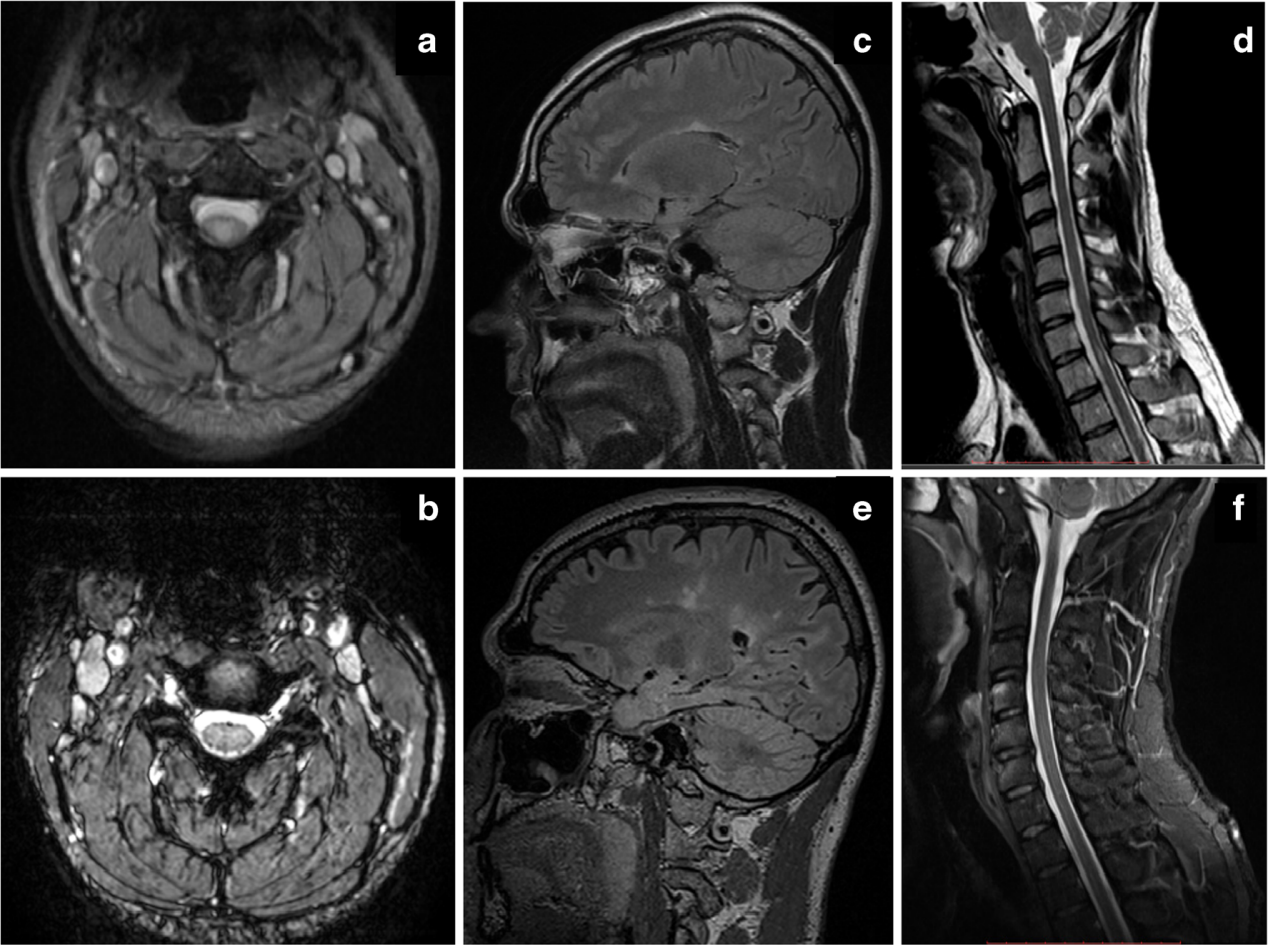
(a) and (b): axial T2-weighted cervical spine MRI of the index case 1, treated with ETN for 8 months for PsA, who was diagnosed with MS on follow-up. (a) At initial presentation, a central posterior demyelinating lesion on C3 level that showed gadolinium enhancement on T1-sequences (not shown). (b) Four years after etanecept discontinuation, disappearance of the lesion. (c)–(f) Brain and cervical spine MRI of the index case 2, with AS and a family history of MS, treated with adalimumab for 36 months, who was also diagnosed with MS. (c) Brain flair and (d) cervical spine T2-weighted sagittal MRI at symptom onset, and (e)–(f) 4 years after anti-TNF-a cessation. Note the increase of cerebral demyelinating lesions, with marked atrophy, and the disappearance of the lesion on C7 level
